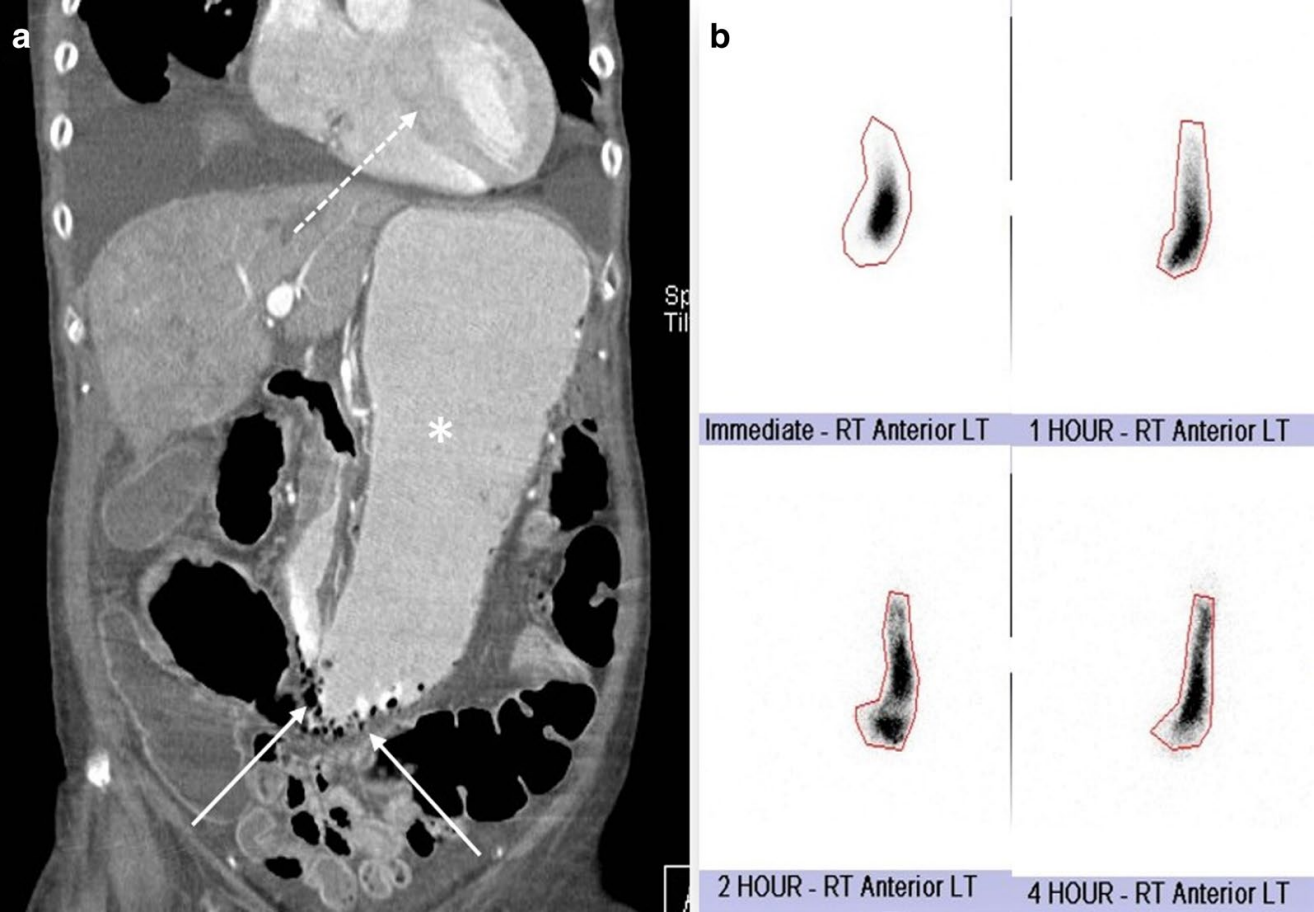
A 56 y/o male with known cardiac amyloidosis, abdominal pain and nausea. a CT of abdomen showed thickening of the left ventricle myocardium related to amyloid deposition (dotted arrow). Additionally, there are gastric distension (asterisk) and pneumatosis (thin arrows). Endoscopic biopsy of the bowel wall revealed amyloid deposition as the underlying cause. b Prior gastric emptying study showed markedly delayed emptying. Gastric emphysema and ischemia were found at surgery
